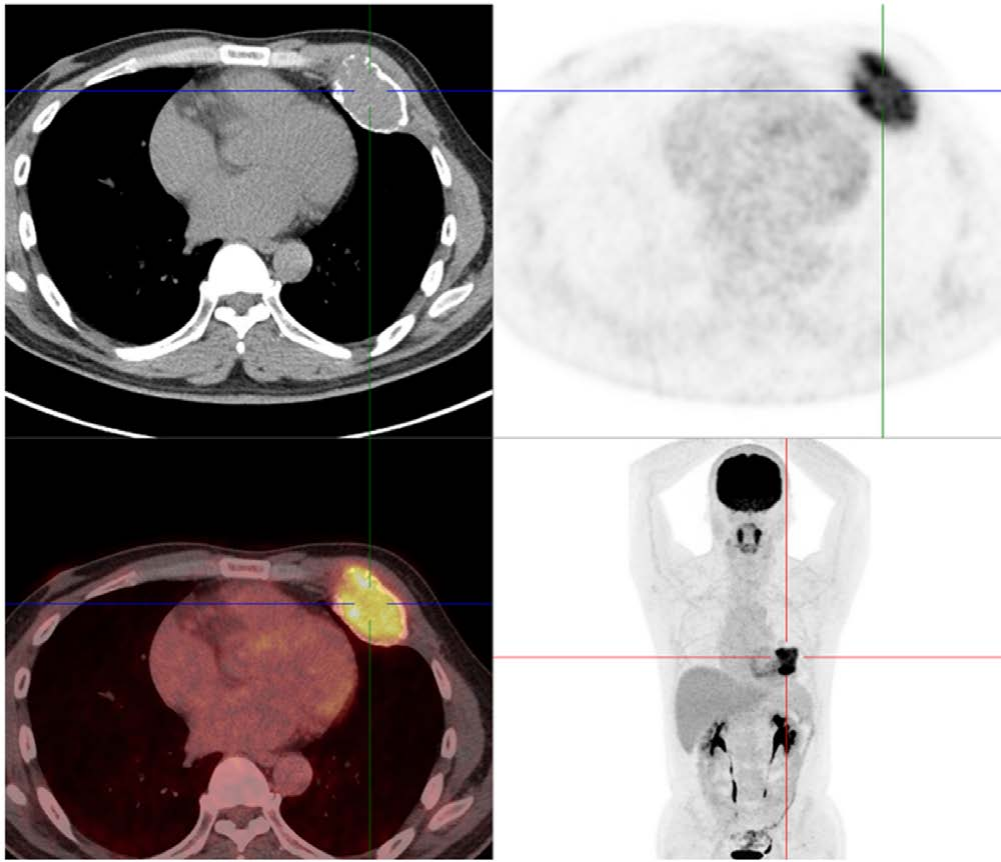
Fluorine-18-FDG PET showed that the size of the mass was about 11 × 11 × 9 cm, several high-density shadows similar to calcified lesions, uneven density, unclear outline of intercostal muscles, widened intercostal space of the fifth and sixth ribs, and the CT value was between 15 and 50. CT = computed tomography, FDG = fluorodeoxyglucose, PET = positron emission tomography.
